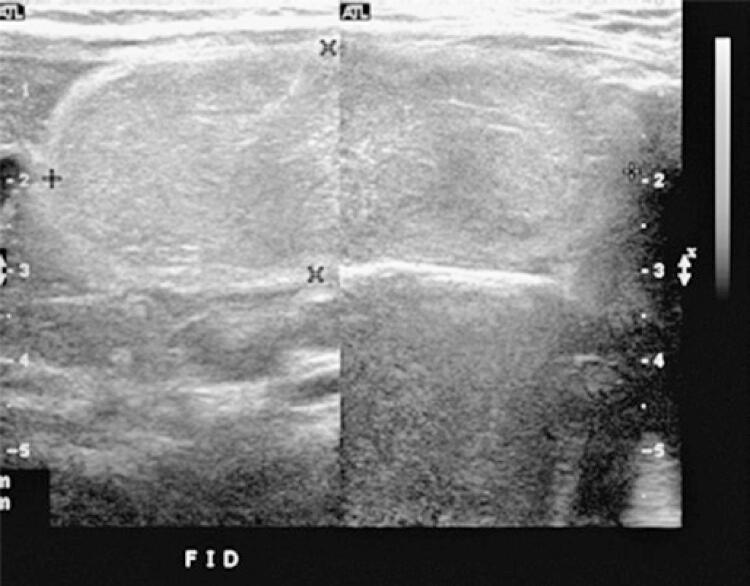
Ultrasonography showing an elongated, well-defined, echogenic and slightly heterogeneous mass, with low flow on Doppler assessment
